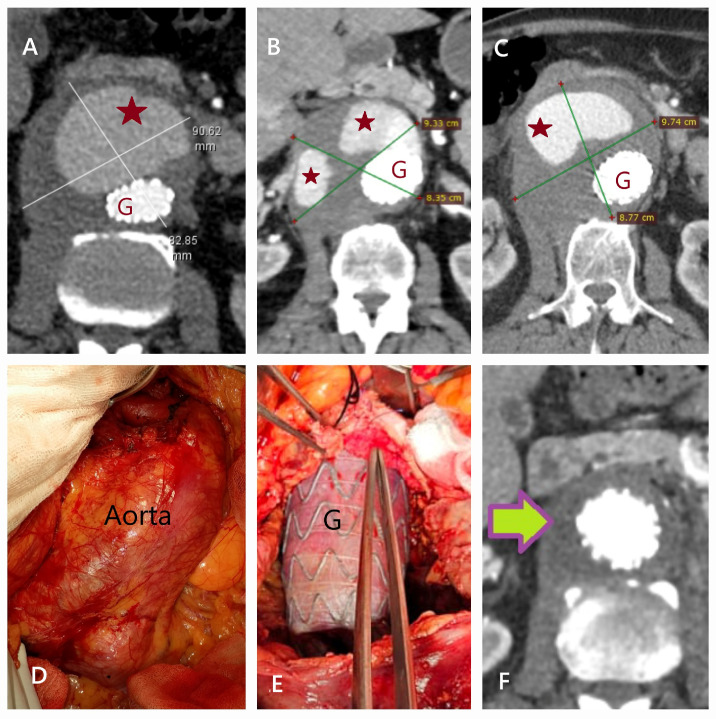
CT and surgical images of Type 1 endoleak. (A–C) CT scans taken at the first, fourth, and sixth months following EVAR in a 70-year-old male patient demonstrate progressive aneurysm enlargement due to a Type 1 endoleak (red stars indicate contrast agent outside the stent graft; G, stent graft). Despite an intervention with a fenestrated tube graft in the fourth month, aneurysm expansion continued. (D,E) During open surgery, the aneurysm sac was opened without cross-clamping using an endovascular occlusion balloon. The anterior leakage site (forceps tip) was repaired with pledgeted sutures, and the aneurysm sac was wrapped around the graft. (F) The patient’s postoperative second-month CT scan (green arrow) is shown. Stent-preserving surgery is a less invasive alternative to stent explantation in managing EVAR-related complications.
